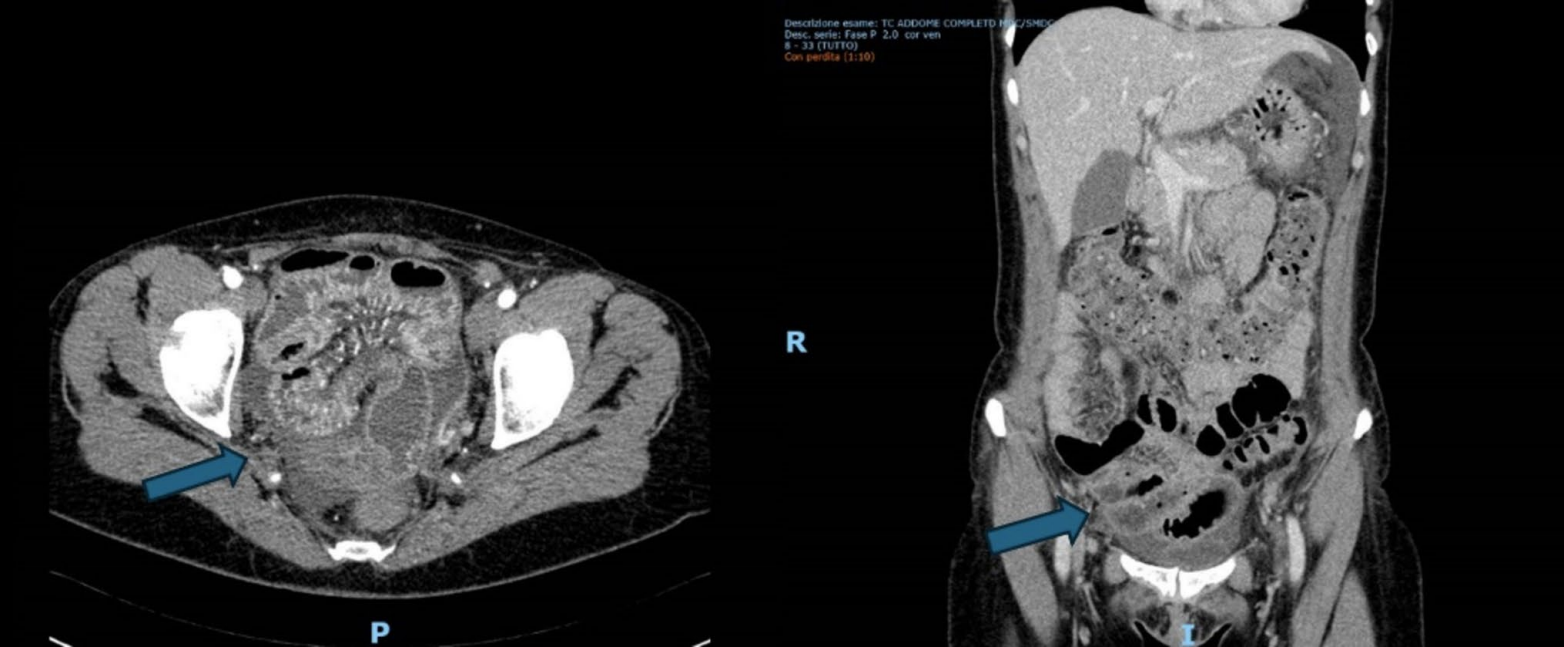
Emergency abdominal CT scan. The arrows showed the “*Distension of the small bowel loops in the pelvis with 15 cm slightly thickened—edematous and hypovascularized small bowel walls as in ischemic ileitis (post-embolic?), in the absence of significant alterations in the canalization of the main tributary vessels”*
